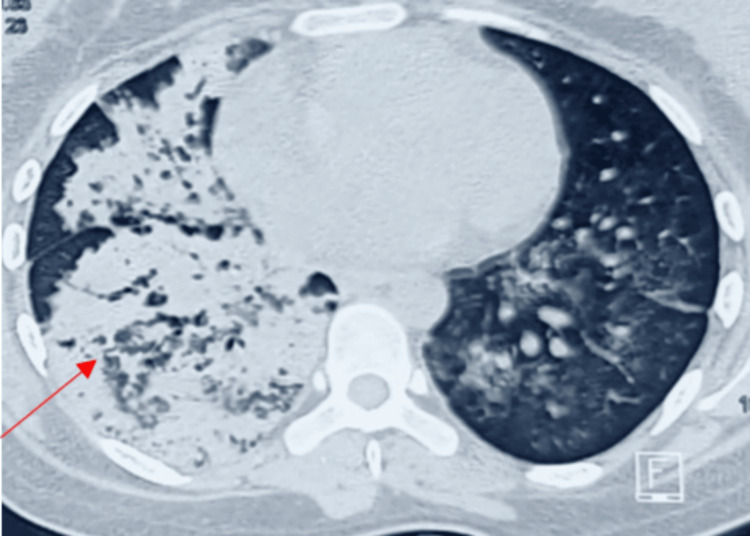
Chest high-resolution computed tomography (HRCT) showing right unilateral diffuse alveolar opacities (red arrow)
